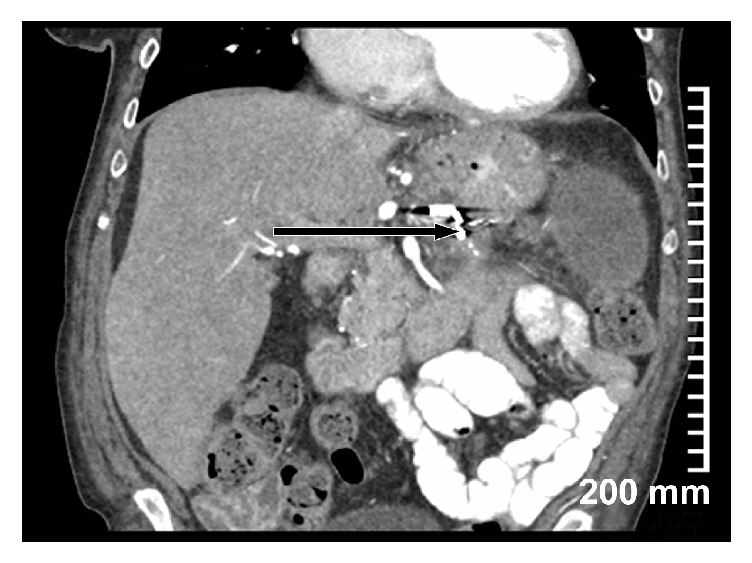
Coronal CT abdomen image showing vascular coils in close proximity of distal duodenum.
